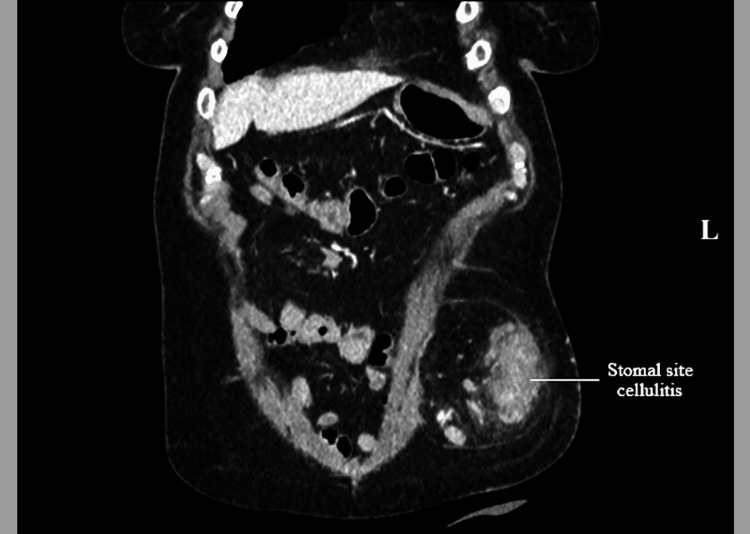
Computed tomography (CT) of the abdomen showing stomal site cellulitis
